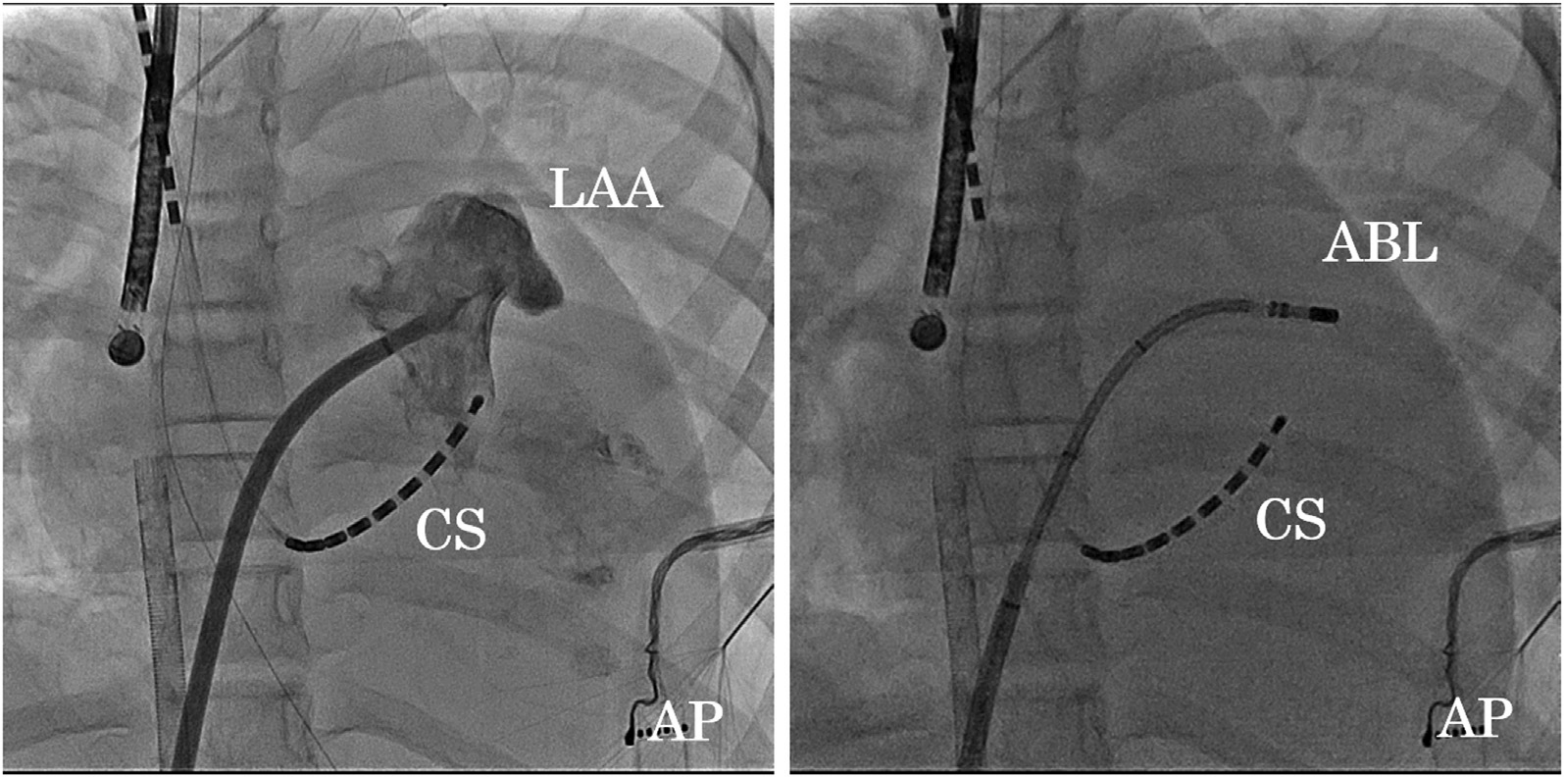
Successful Ablation Site of LAA (Left) Cardiac angiography of the LAA. (Right) AT stopped after the catheter ablation. ABL = ablation catheter; AP = anteroposterior radiographic view; AT = atrial tachycardia; CS = coronary sinus; LAA = left atrial appendage.
